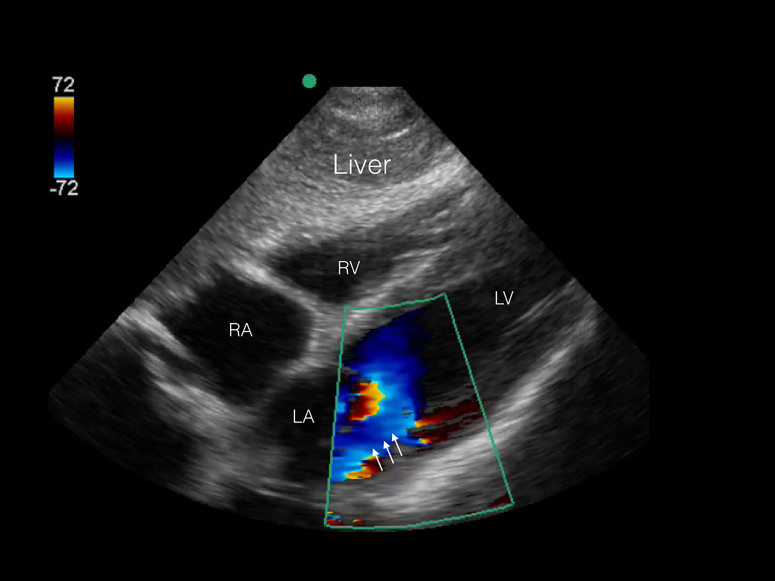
A subxiphoid four chamber view of the heart with color Doppler demonstrates a severe mitral regurgitation jet (arrows). *RA*, right atrium; *RV*, right ventricle; *LA*, left atrium; *LV*, left ventricle.
